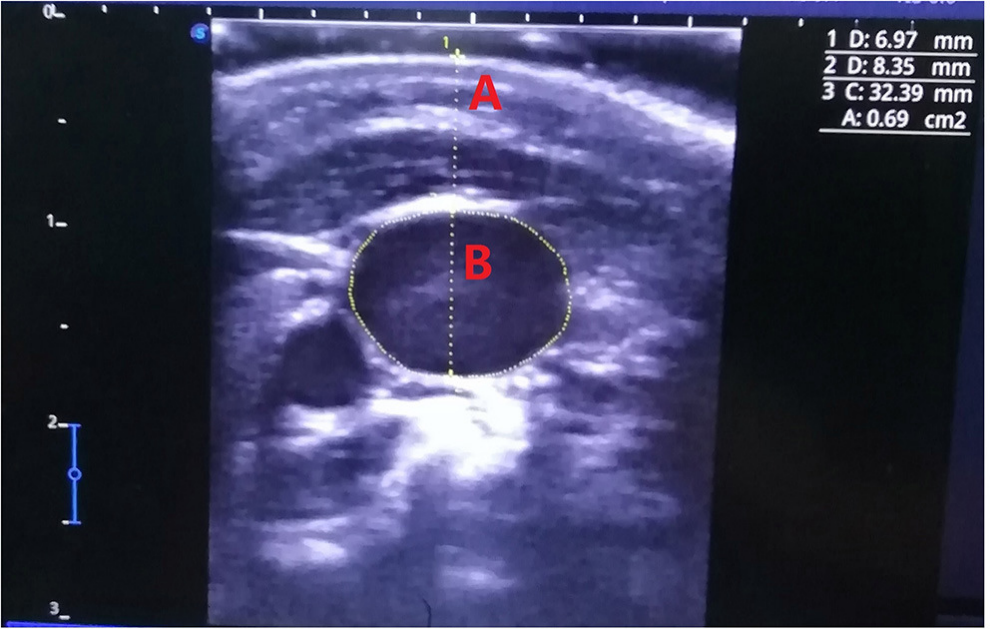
Ultrasound measurement of internal jugular veins.
